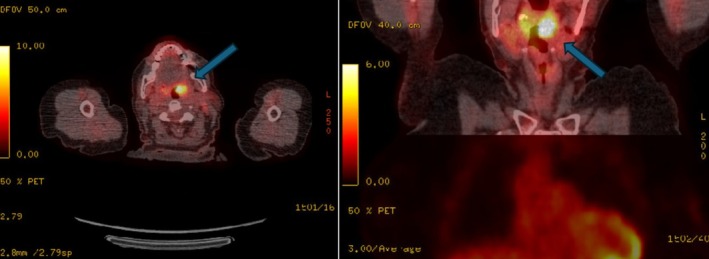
Staging PET‐CT showing axial and coronal views with fluorodeoxyglucose (FDG) uptake in the left tonsillar region.
